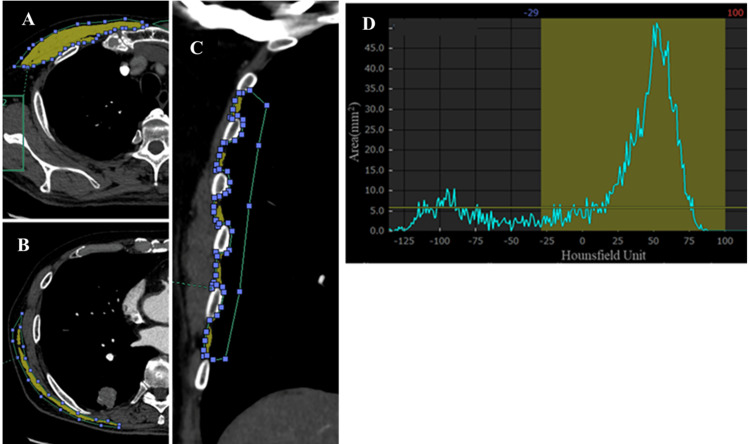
Method for measuring respiratory muscle cross-sectional areas (CSAs) (A) In the transverse section image, the region of interest (green line) is drawn at the outermost edge of the pectoralis muscles (pectoralis major and pectoralis minor) in the anterior thoracic region. (B) In the transverse section image, the region of interest (green line) is drawn at the outermost edge of the latissimus dorsi muscle at the level of the inferior border of the eighth thoracic vertebra. (C) In the coronal image, the region of interest (green line) is drawn at the outermost margin of the third to eighth intercostal muscles bilaterally. (D) CT histogram analysis automatically measured the CSAs and attenuation of each muscle (CT attenuation ranged from -29 to 100 HU). Each automatically measured muscle area is in the yellow range.
